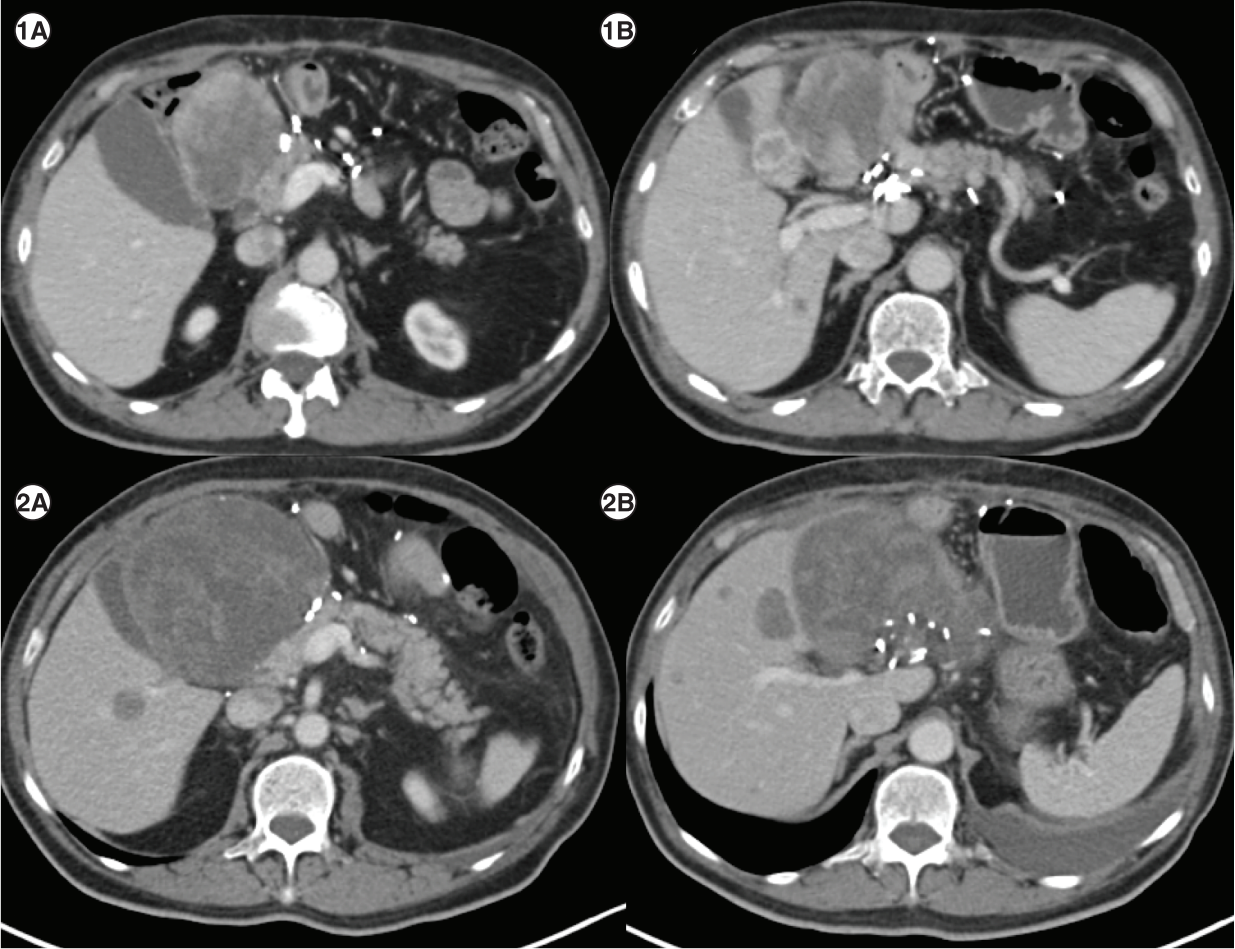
Response to imatinib in case 5. (1A) Axial computed tomography abdomen of patient E showing a heterogenous mass measuring 7.1 × 6.6 cm in the surgical bed in the gastro-hepatic region and a hypodense lesion in segment IVB of liver (1B) measuring1.5 × 1.8 cm. (2A) Axial CT abdomen of first follow-up scan showing a heterogenous mass measuring10.2 × 10.7 cm in the surgical bed in the gastro-hepatic region and a hypodense lesion in segment IVB of the liver measuring 2.9 × 2.8cm (2B), suggestive of disease progression.
